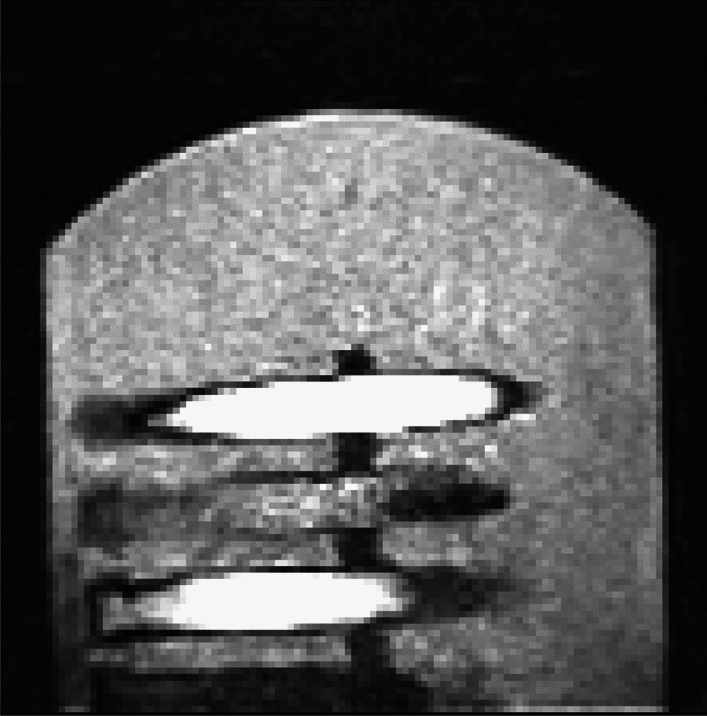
64 mT T1-weighted Coronal image using the domed top with the sample tubes not aligned
